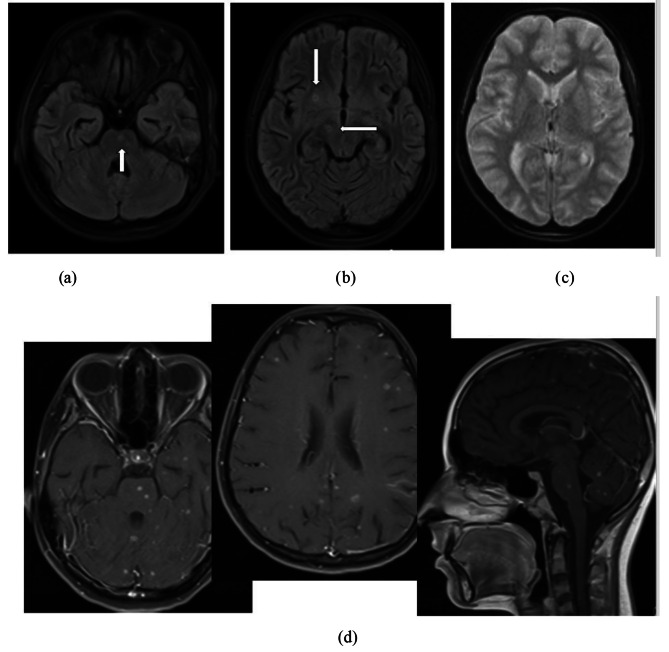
Brain MRI without contrast: 4-a & 4-b) Flair sequence: some central hypo-intense with hyper-intense rim nodules in the pons, right cerebral peduncles and lentiform nucleus (white arrows). 4-c) DWI/ADC: no restricted diffusion. 4-d) Contrast–enhanced MRI: Diffuse Multiple rim-enhancing nodules in both cerebral, cerebellar hemispheres and brainstem. These findings are suggestive of caseating tuberculomas with tuberculous meningitis. DWI: Diffusion-weighted imaging – ADC: Apparent diffusion coefficient
